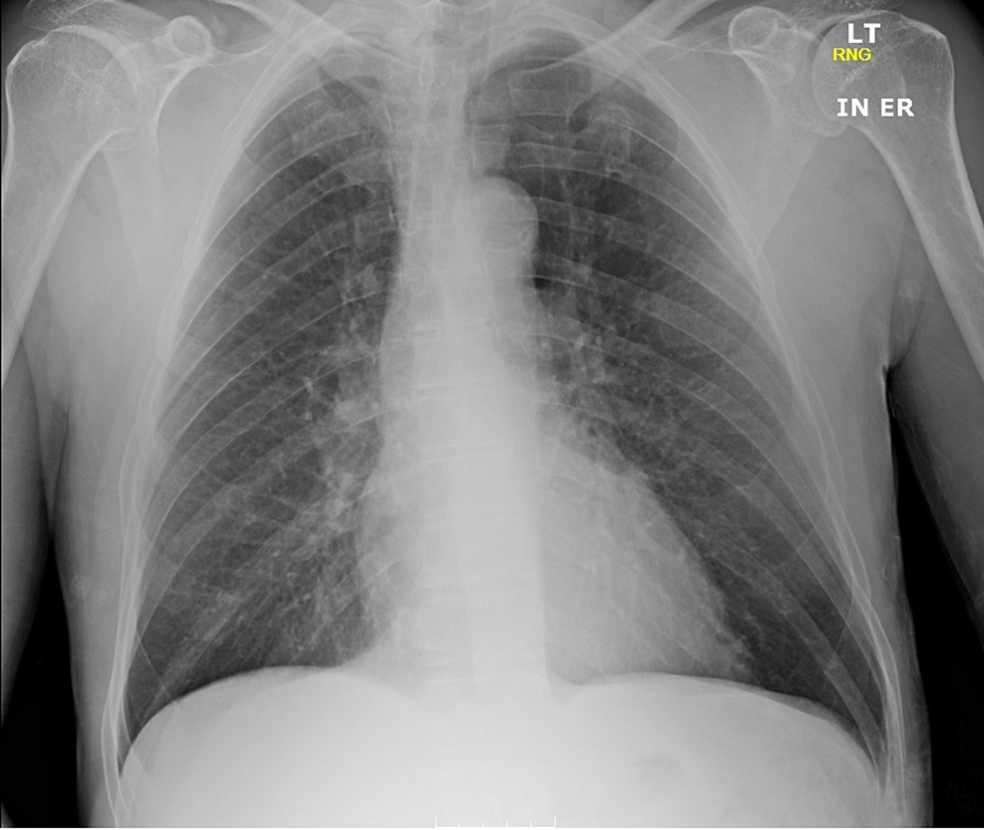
Chest X-ray A single anteroposterior portable chest X-ray was obtained on admission. No acute intrathoracic processes were observed. Mild interstitial prominence in the lungs possibly related to chronic pulmonary disease was documented
